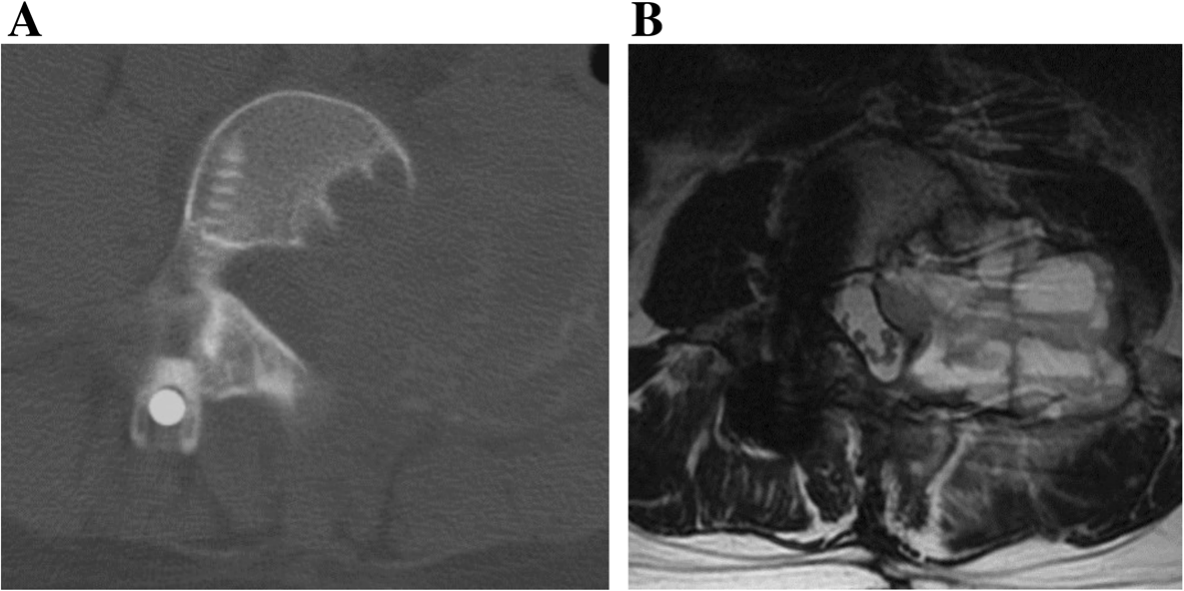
a Axial CT of L4 obtained 3 months after the 2^nd^operation. The region extended from the epidural space to the paravertebral area. b The same level on T2 axial MRI showed a fluid-fluid level containing lesion. Re-recurrence was suspected
